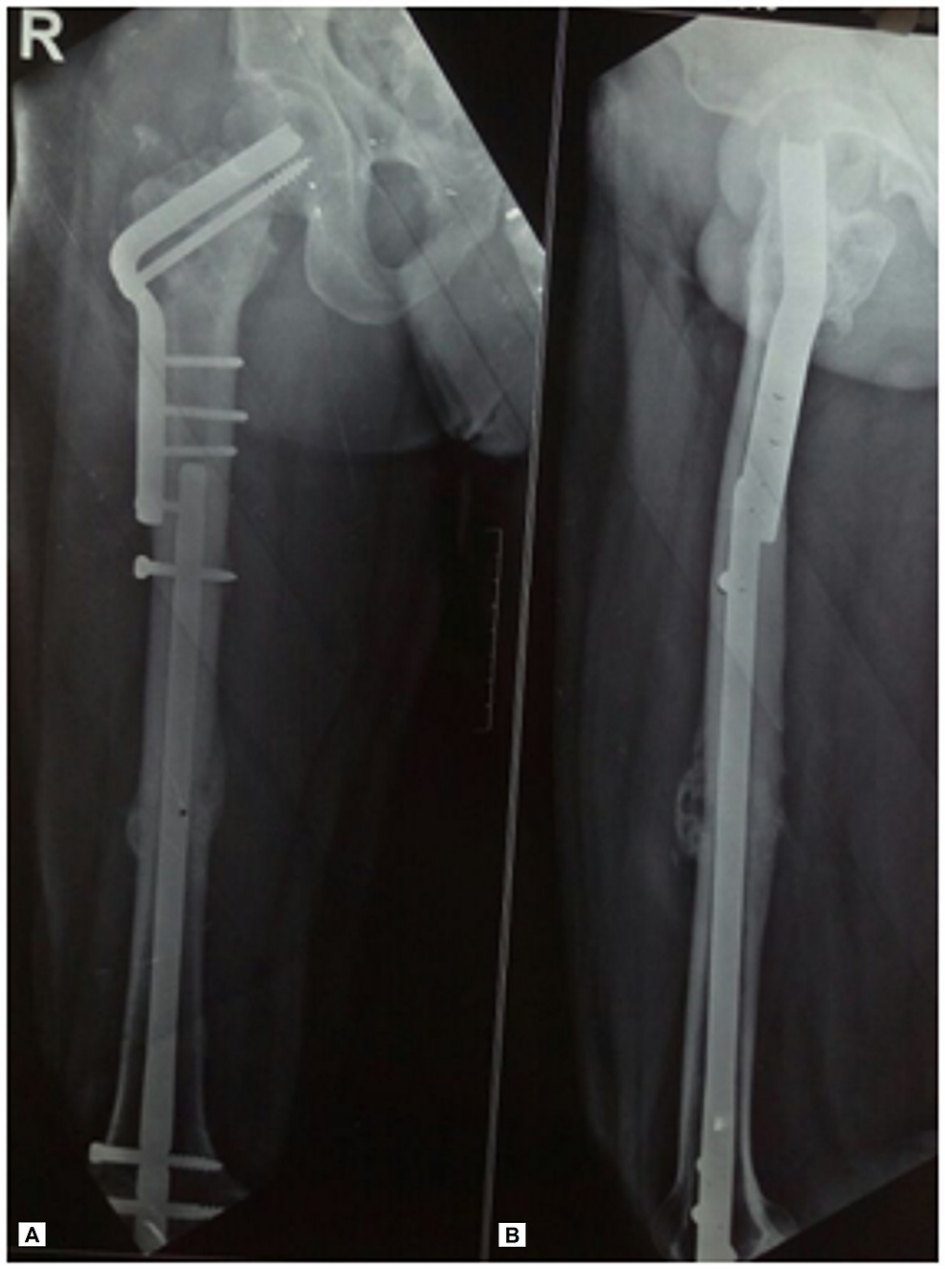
(A, B) Radiographs taken five months postoperatively showing union at the femoral neck and osteotomy site
